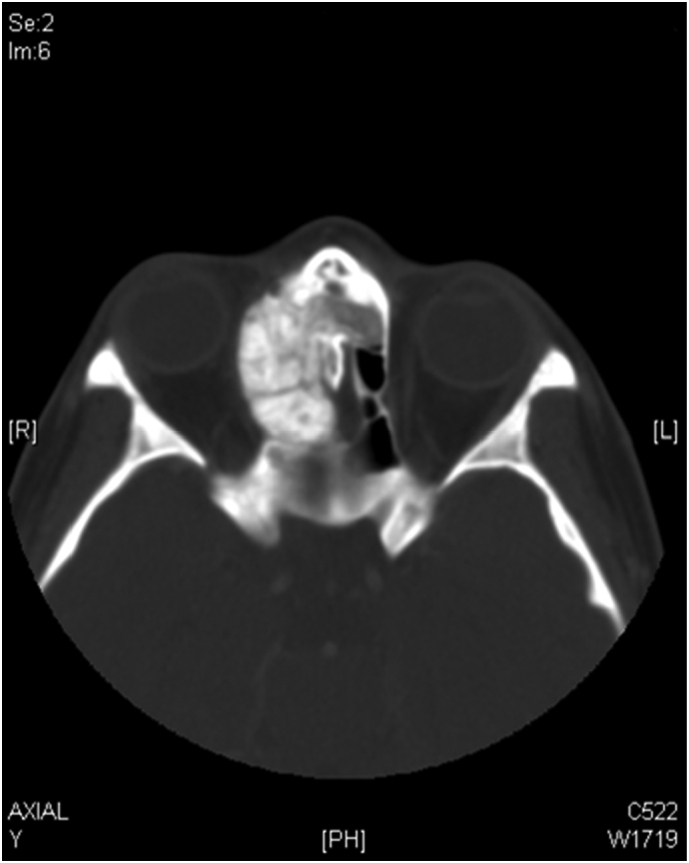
Paranasal axial CT scan shows right ethmoid sinus and nasal cavity mass with orbital and intracranial involvement.
